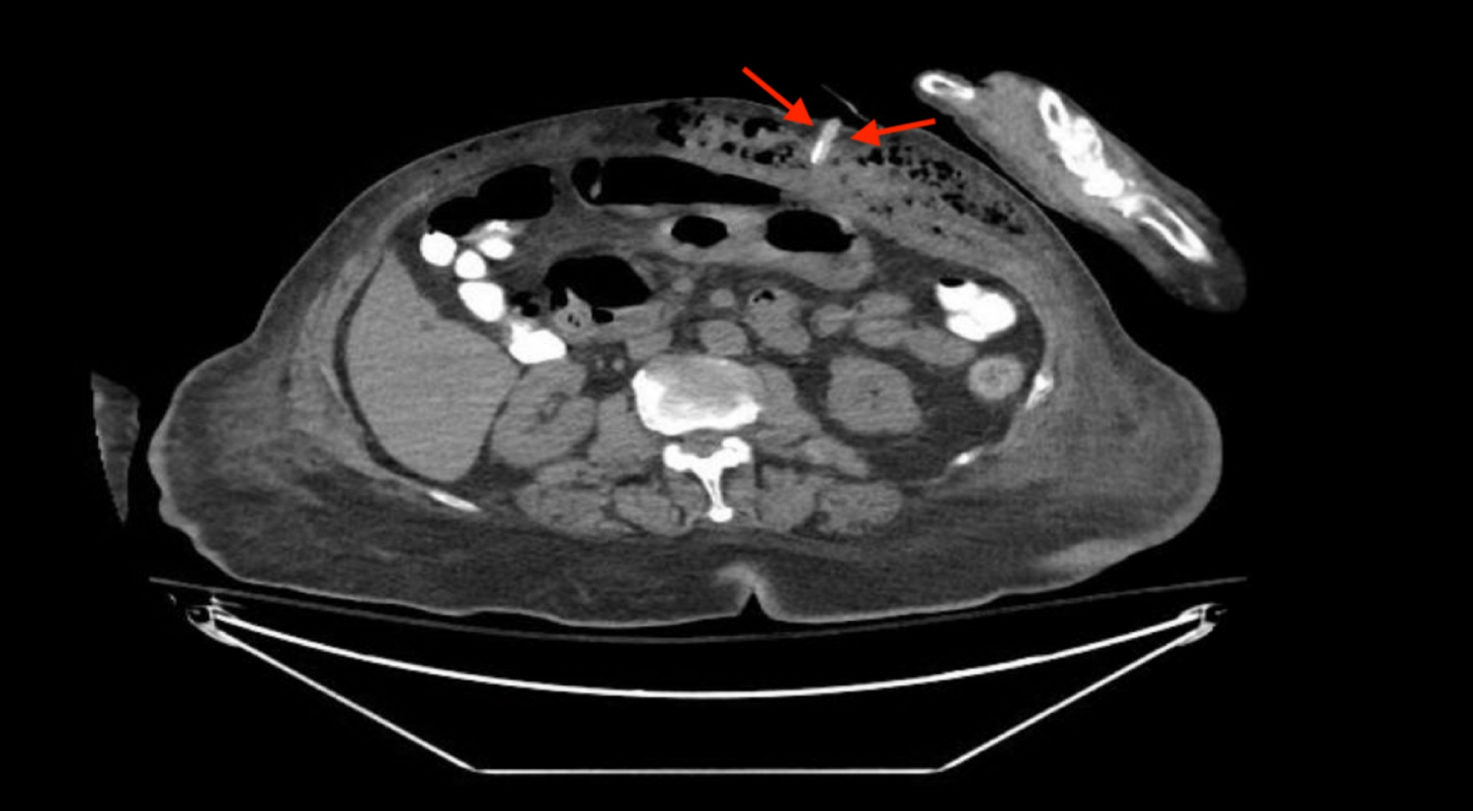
Computed tomography (CT) of the abdomen which shows the misplaced gastric tube in the upper abdominal wall at the level of the gastric antrum
